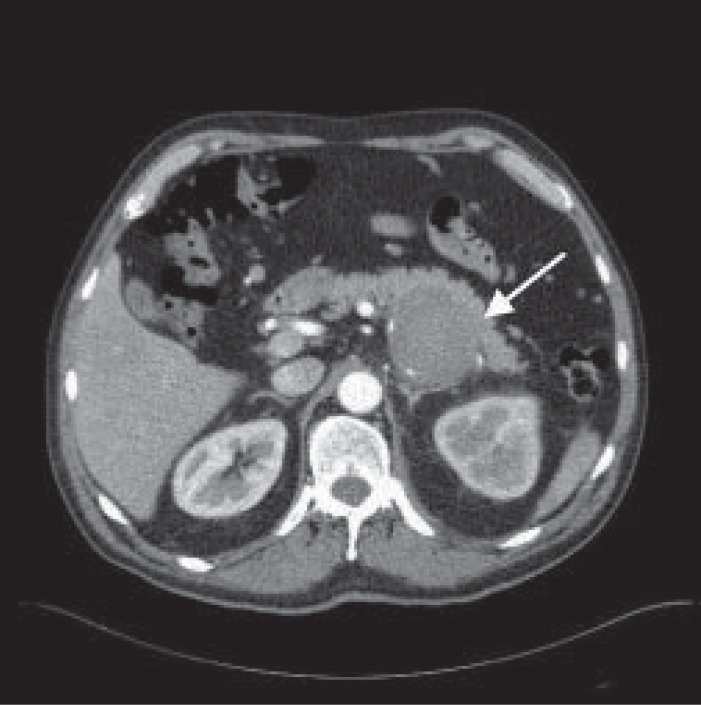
Follow up CT angiogram shows no flow in the aneurysm sac (arrow).
